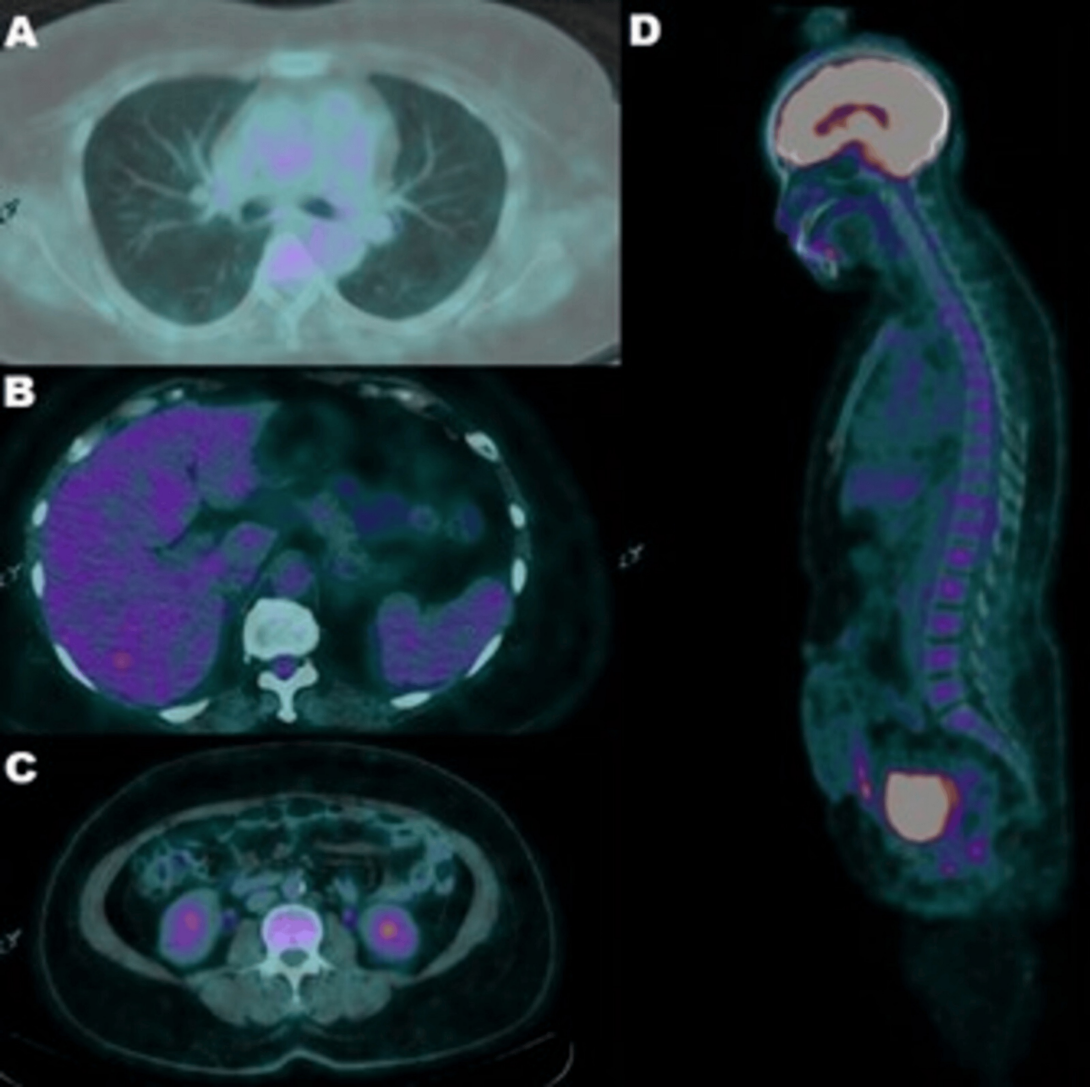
Continued baseline [18F] fluorodeoxyglucose positron emission tomography/computed tomography (PET/CT) performed for the detection of metastasis (A) thoracic transaxial PET/CT, (B) hepatic transaxial PET/CT, (C) renal transaxial PET/CT, and (D) head-abdomen sagittal PET/CT.
